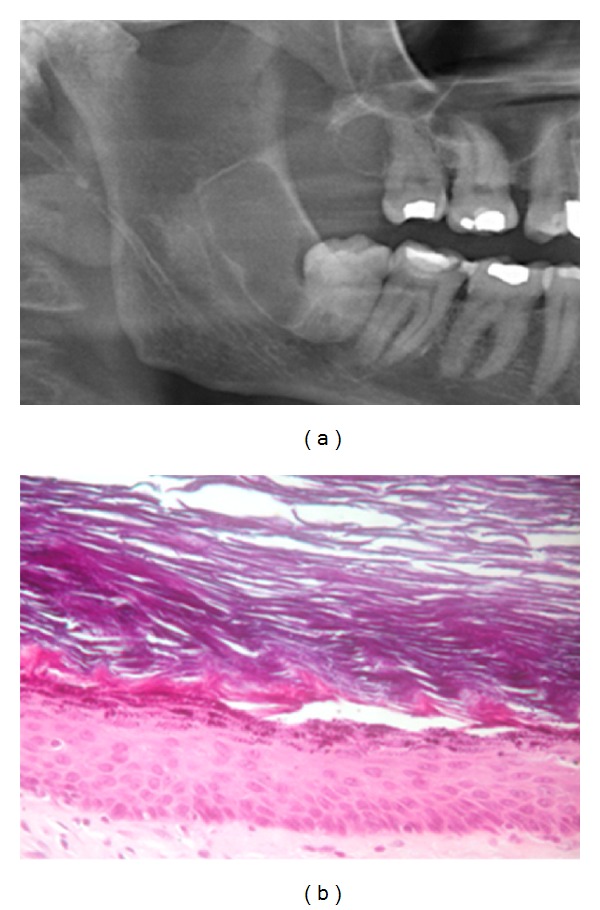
(a) Initial orthopantomography. (b) Fibrous connective tissue with a stratified epithelium with hyperorthokeratosis lining.
